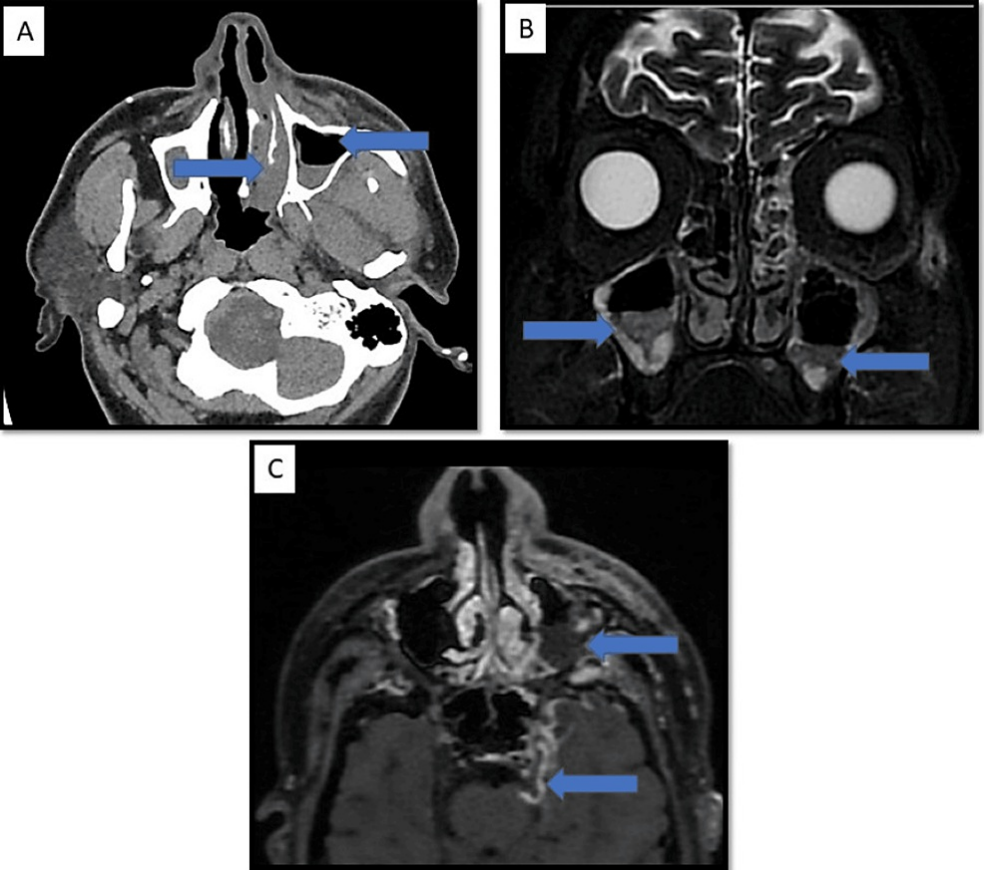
Intracranial involvement: case 1 A 33-year-old male of post-COVID status presented with left-sided facial pain and swelling. Image A-AXIAL CT image shows mucosal thickening involving the left middle turbinate and mild mucosal thickening with fluid level in the left maxillary sinus. The patient was classified as stage 2 ROCM and sent for a biopsy. The biopsy was negative for an invasive fungal infection. However, the same patient underwent MRI imaging 10 days later. Image B: Coronal T2 STIR image shows evidence of significant mucosal thickening and fluid collection in bilateral maxillary sinuses (L>R), left ethmoid air cells, and also in the left frontal sinus. Image C: Axial T1 post-contrast image shows involvement of the left Meckel’s cave and trigeminal nerve up to the pre-pontine cistern. These findings are consistent with perineural infiltration and cranial nerve involvement.
